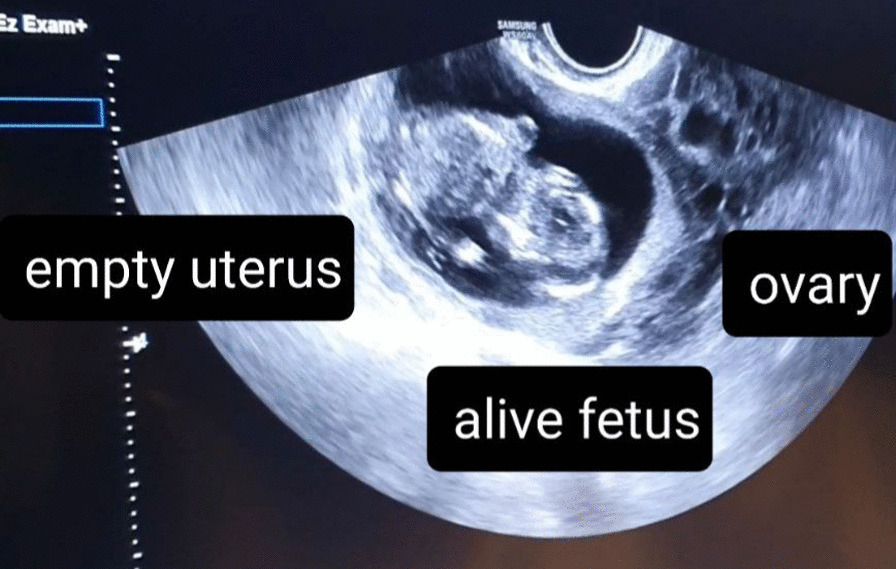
Evidence of ovarian ectopic pregnancy (EP) in the Transvaginal sonography (TVS)
